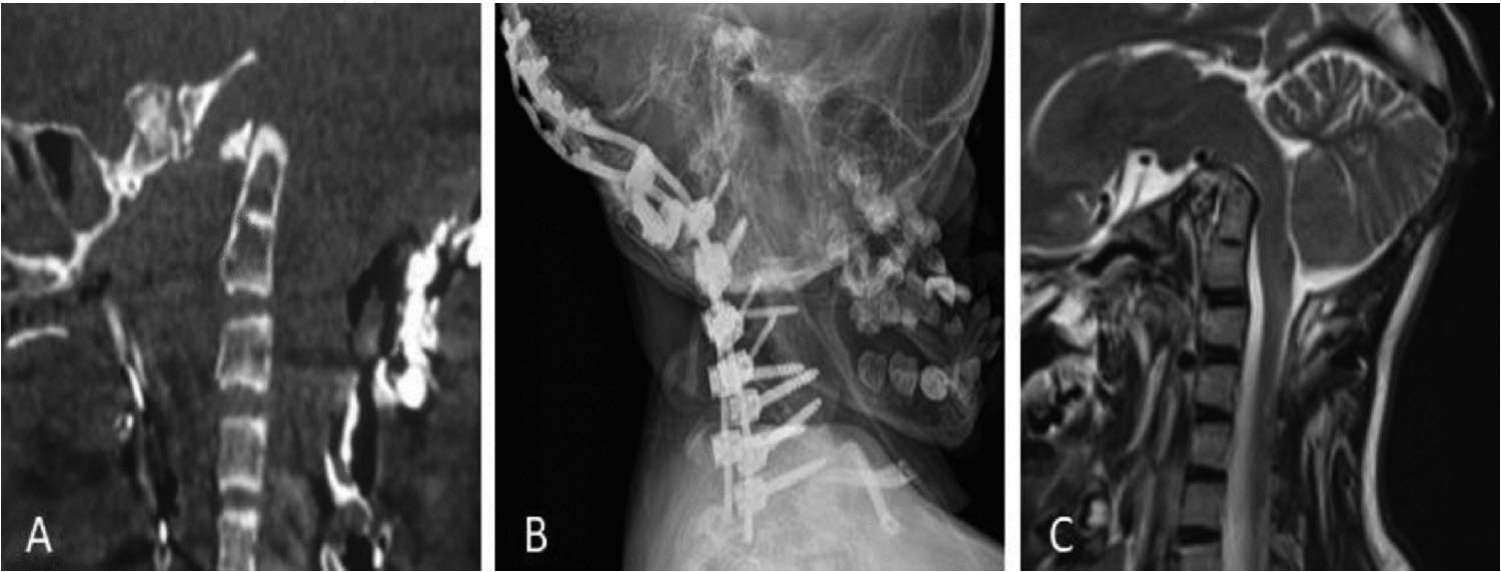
Postoperative and follow-up images. A Immediate postoperative CT scan showing that the deformity correction obtained with the Halo is preserved. B Cervical X-rays at 6 months follow-up, showing a stable reduction of the deformity. C MRI at 6 months follow-up, showing the posterior fossa decompression resulting in a reduced mass effect on the brainstem and restored CSF circulation at the CVJ
